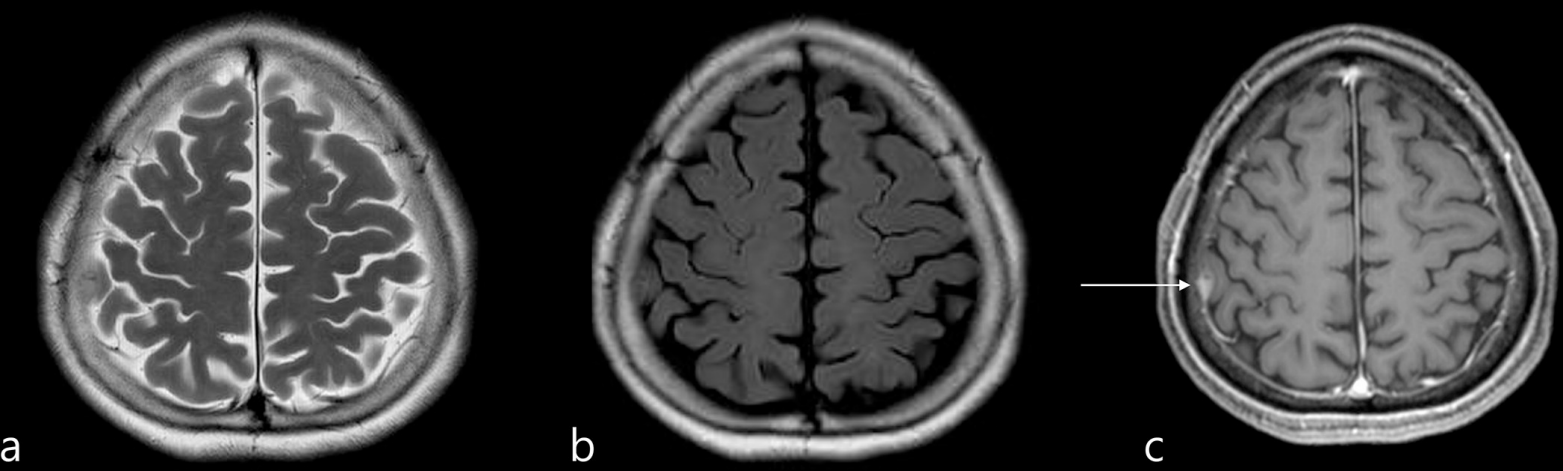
(a, b, c) Images in a 66-year-old female patient with a small extra-axial mass at the right parietal convexity suggestive of meningioma (arrow), which became noticeable with the aid of contrast enhancement study. (a, T2 weighted image; b, FLAIR; c, contrast-enhanced T1 weighted image).
